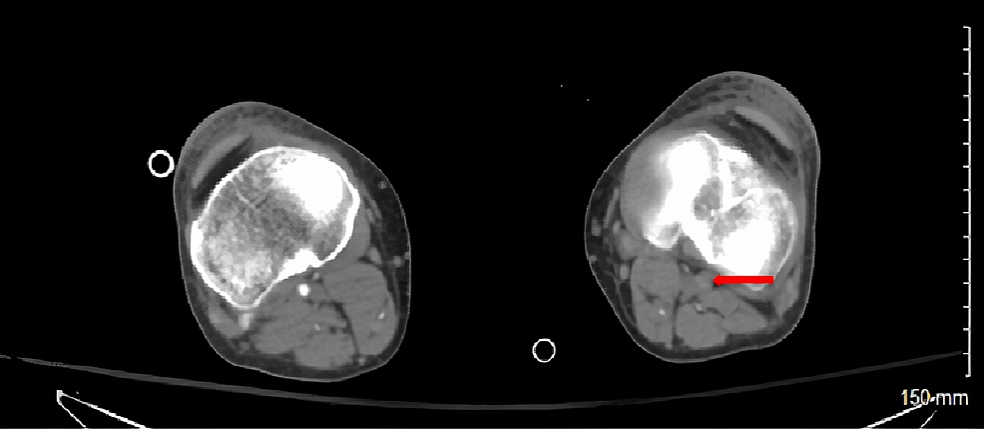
CT angiogram of lower extremities identifying left popliteal occlusion (red arrow).
